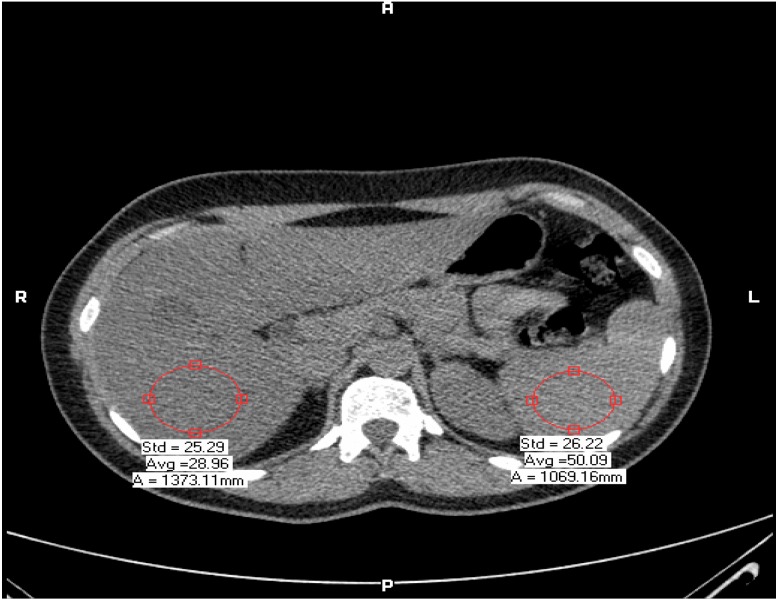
Example of fatty liver diagnosed by CT: liver minus spleen density > −10 Hounsfield units (HU).
